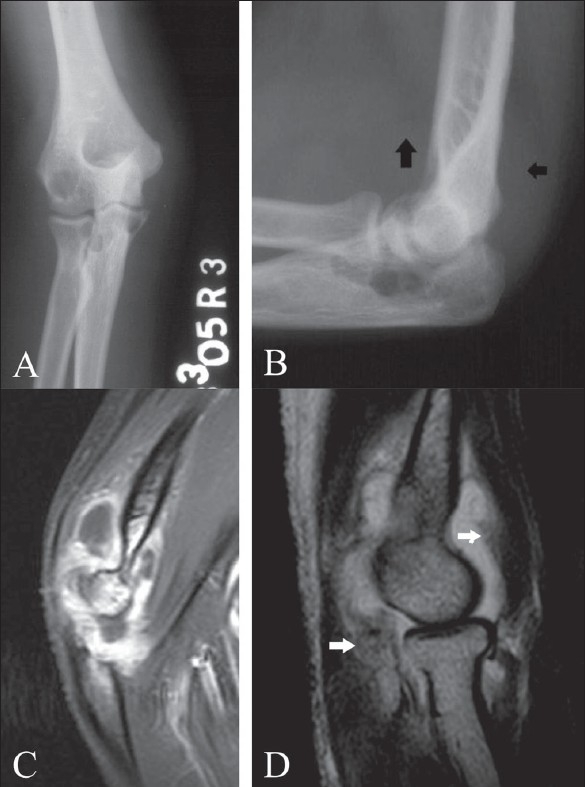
Tuberculous arthritis of the right elbow. Frontal (A) and lateral (B) plain radiographs demonstrate multiple osteolytic lesions within the ulna, radius and radial condyle. Note also joint effusion with displacement of the peri-articular fat pads of the elbow (arrows). Sagittal, contrast-enhanced, fat-suppressed T1W MRI (C) shows peripheral enhancement of the synovium. Note also enhancement of the adjacent bone marrow. Sagittal, fat-suppressed T2W MRI (D) in another patient shows joint effusion and a relatively hypointense signal of the thickened synovial tissue (white arrows)
